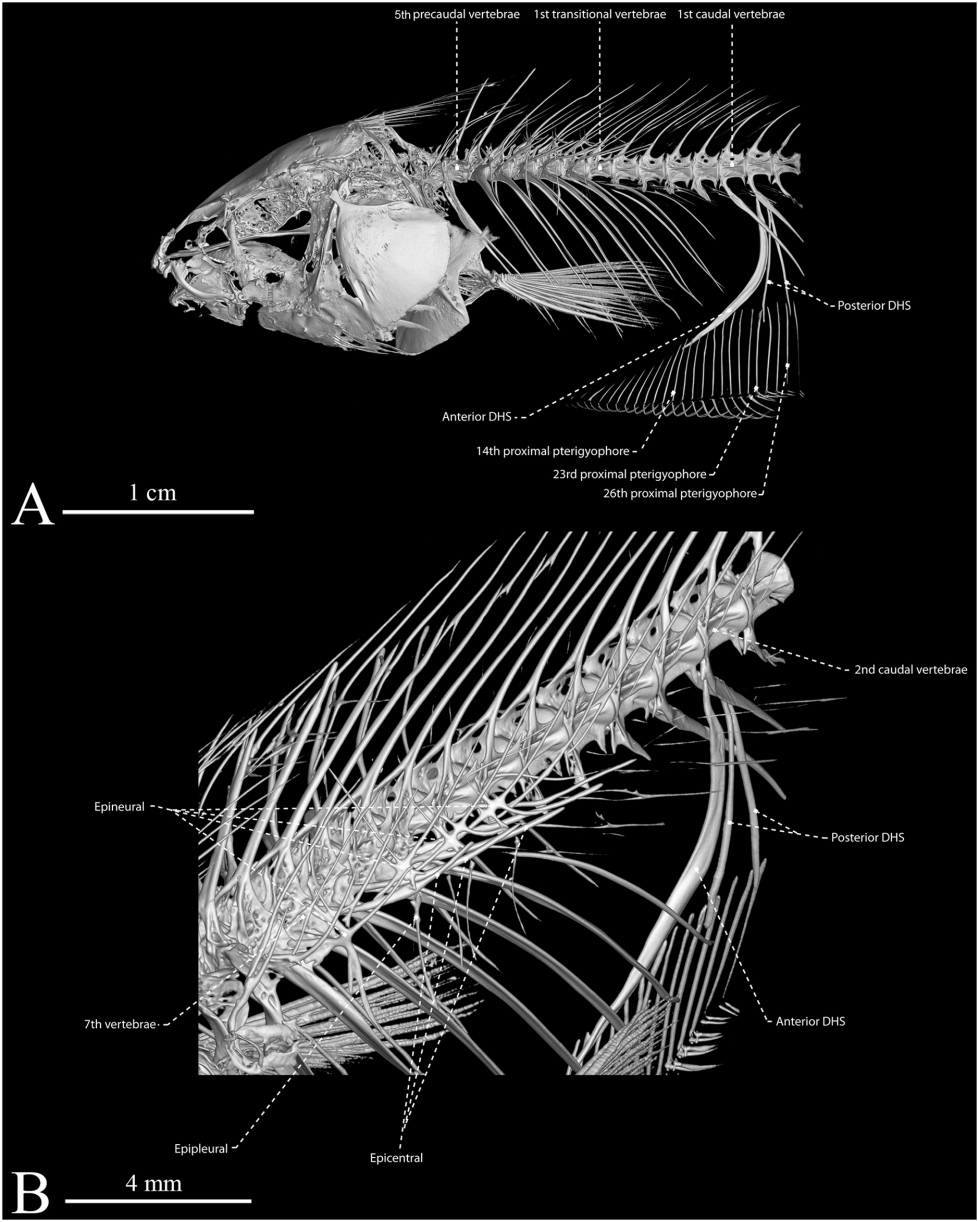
Computed tomography renderings of anterior portion in left lateral (A) and right inverted oblique (B) views of *Eigenmannia sirius*, MZSUP 121668, holotype, 127.5 mm *L*_EA_.
